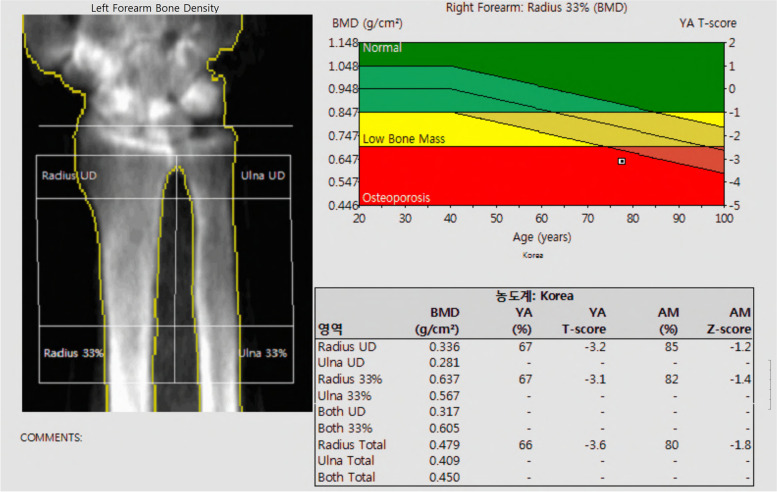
Dual-energy X-ray absorptiometry image of the left forearm. Two regions of interest (ultradistal radius and one-third radius) were included in this study. Radius UD = ultradistal radius, radius 33% = distal one-third radius. The image should include 2 cm of diaphysis over one-third of the forearm and a part of the carpal bones [29]
